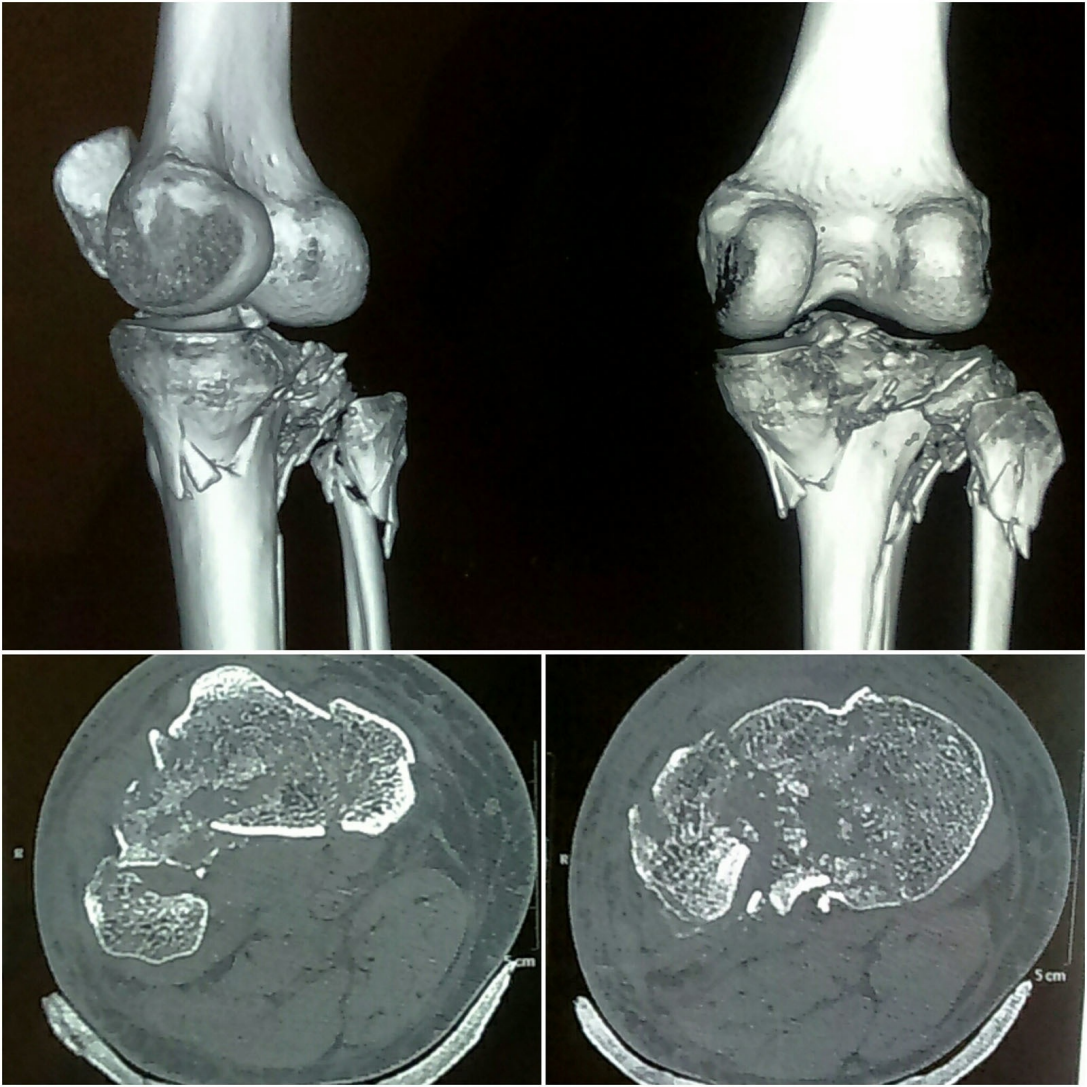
CT scan images depicting the fracture morphology.
